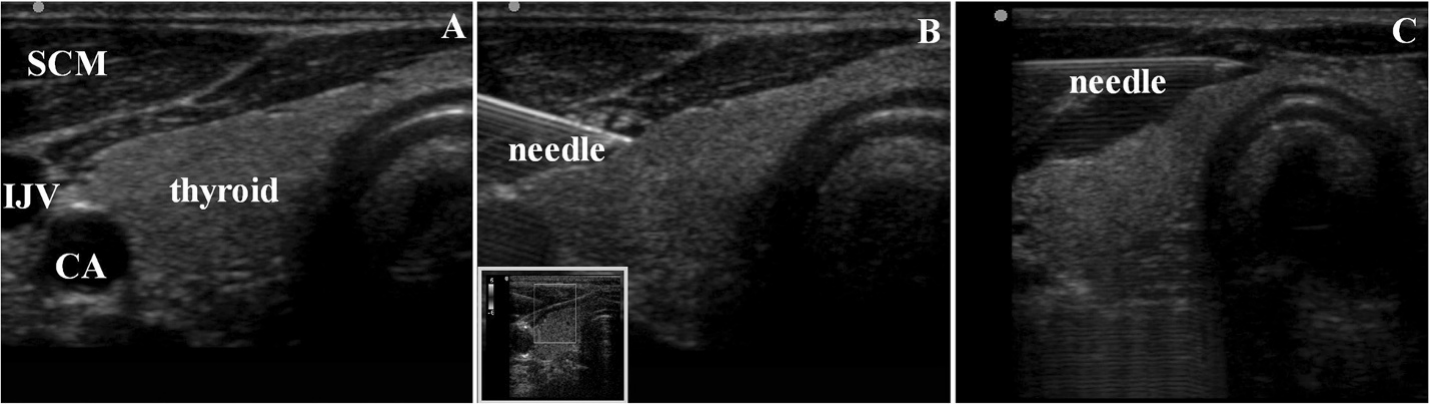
The ultrasound-guided capsule-sheath space block (CSSB) in imaging study: ultrasonogram of the thyroid gland before and after local anesthetic injection. (A) The juncture of internal jugular vein and thyroid gland was identified and placed in the center of the optimal image with minor adjustment of scanning planes; (B) In-plane view of a block needle inserted under color Doppler guidance; (C) Intermittent changes in needle direction to maintain a uniform distribution of contrast medium, especially at isthmus of thyroid. CA, carotid artery; IJV, internal jugular vein; LA, local anesthetics; SCM, sternocleidomastoid muscle.
